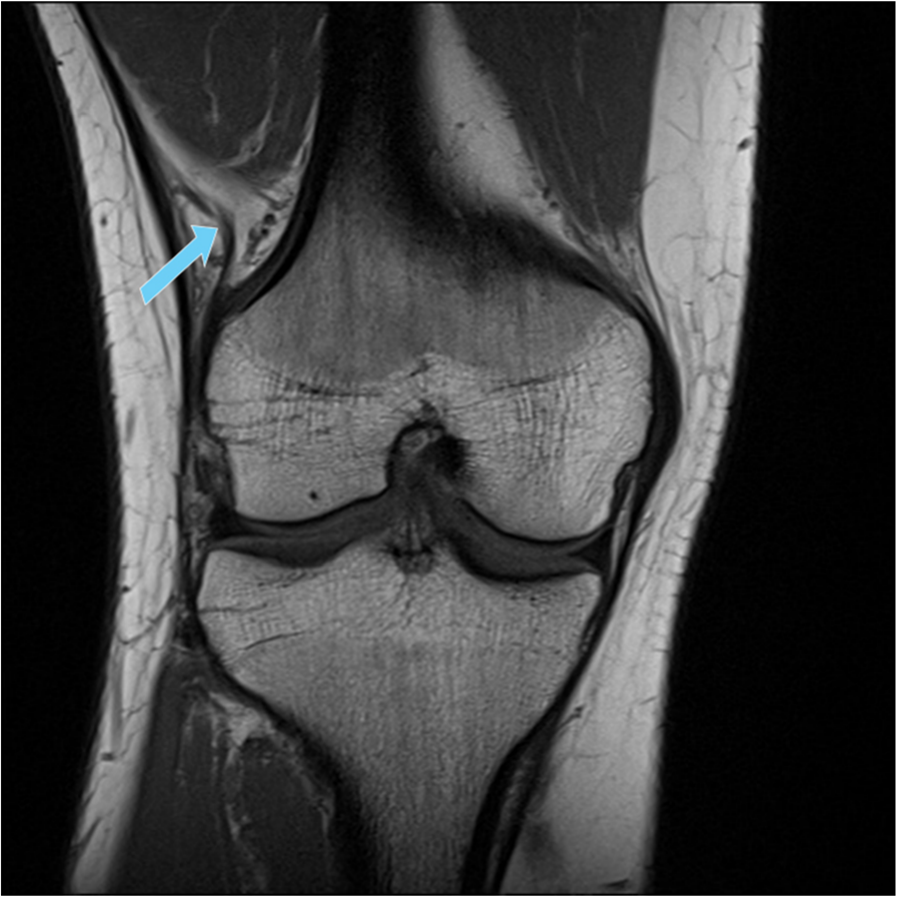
Deep attachments of the iliotibial tract (ITT) to the distal femur (blue arrow) as seen in 60.6% of the examined cases (coronal T1-weighted sequence)
